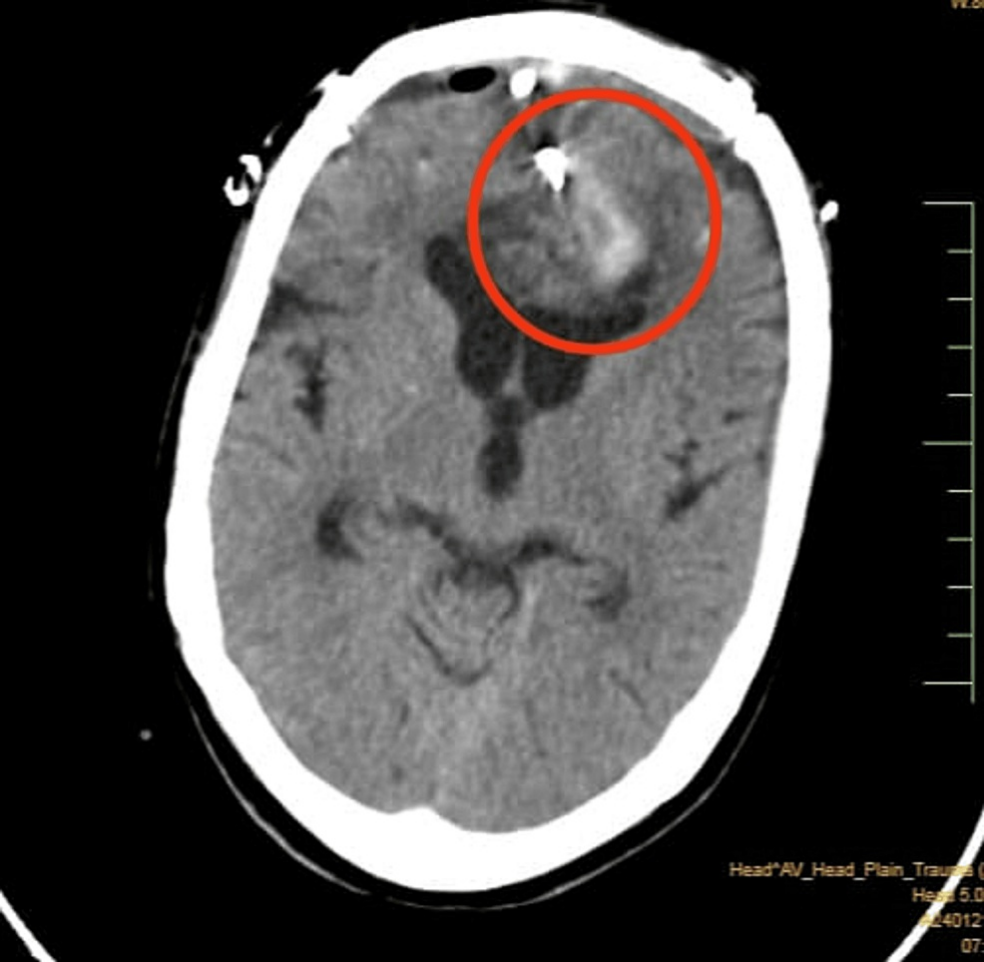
Post-surgery A CT brain shows the plaque calvaria defect.
